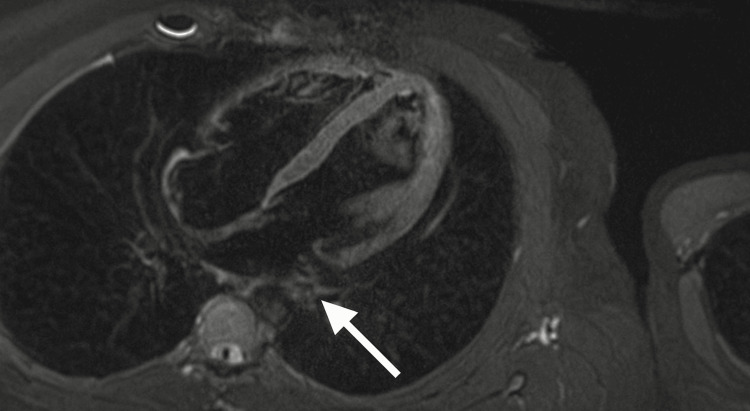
Cardiac magnetic resonance imaging (MRI), T2-weighted four-chamber view Useful for assessing myocardial edema. No active myocardial edema is seen, supporting the absence of acute inflammation (arrow).
